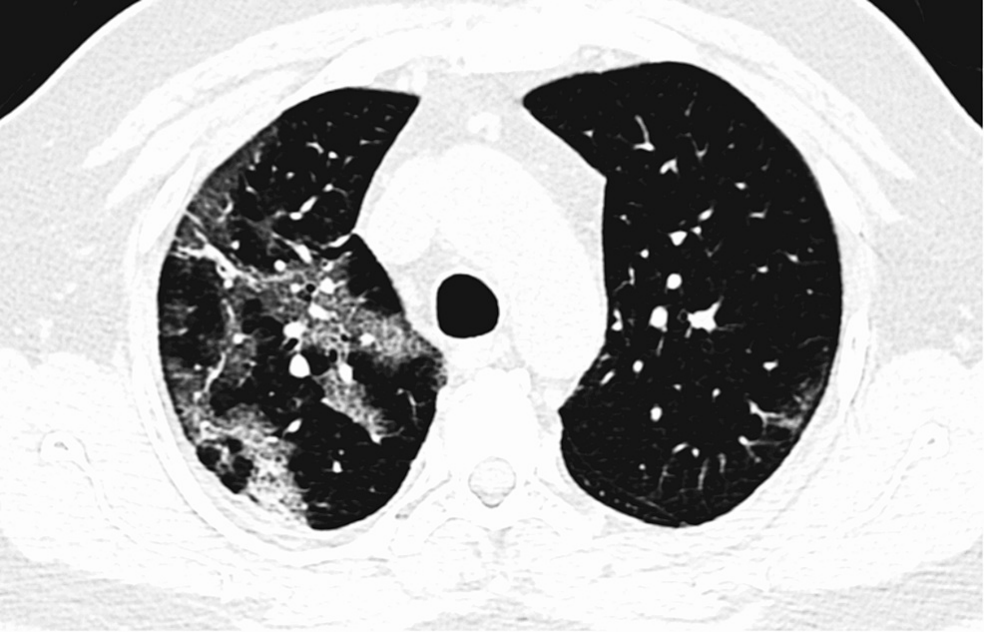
Axial CT section of the chest showing the “crazy-paving” appearance of ground-glass opacities with intralobular septal thickening in a patient with COVID-19 pneumonia
